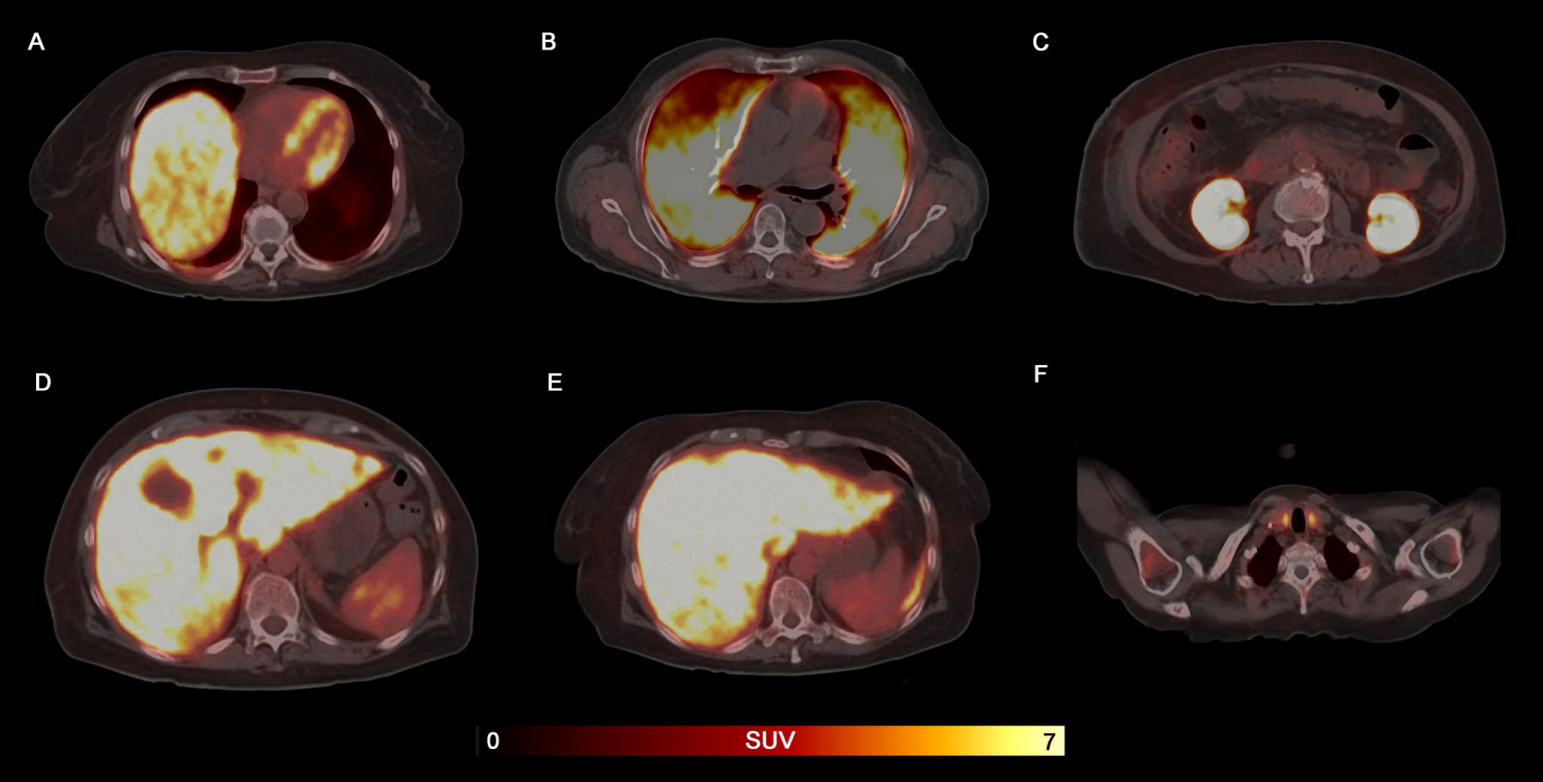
Representative ^11^C-PIB PET/CT images from subjects with biopsy-proven active systemic amyloidosis. Axial fused ^11^C-PIB PET/CT images confirmed diffuse pathological uptake in heart (A), lung (B), kidney (C), spleen (D), liver (E), and thyroid (F)
